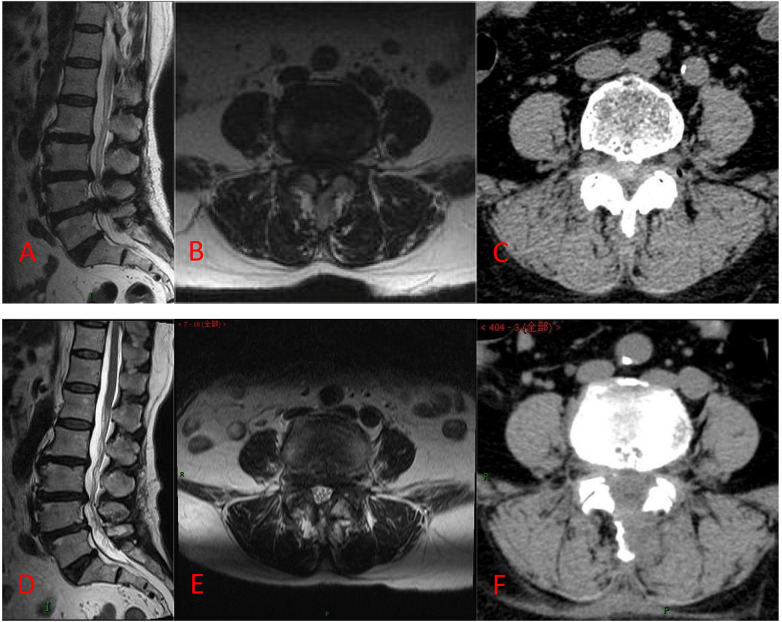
Images from a patient treated with the UNSES technique. (A–C) Preoperative MRI and CT show a disc herniation with spinal canal stenosis at the L4–5 segment; (D–F) postoperative MRI and CT show the disc herniation was excised and the spinal canal was adequately decompressed.
